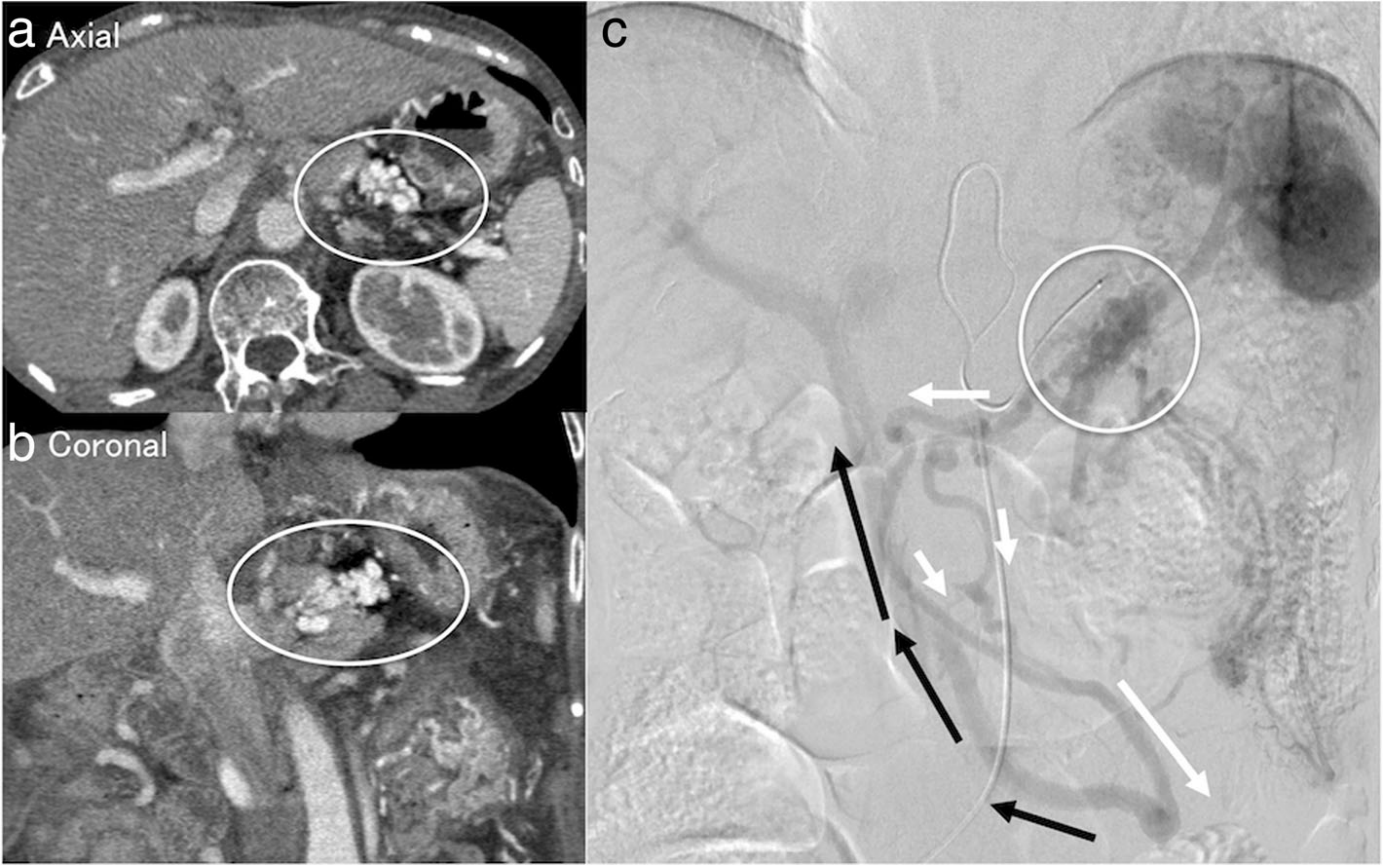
a, b Computed tomography image showing pancreaticojejunostomy varices. c Image of digital subtraction arterial portography from the splenic artery. The flow of splenic vein drain into the varices around the pancreaticojejunostomy (white circle) and finally toward the superior mesenteric vein (black arrow) via the elevated jejunal vein (white arrow)
